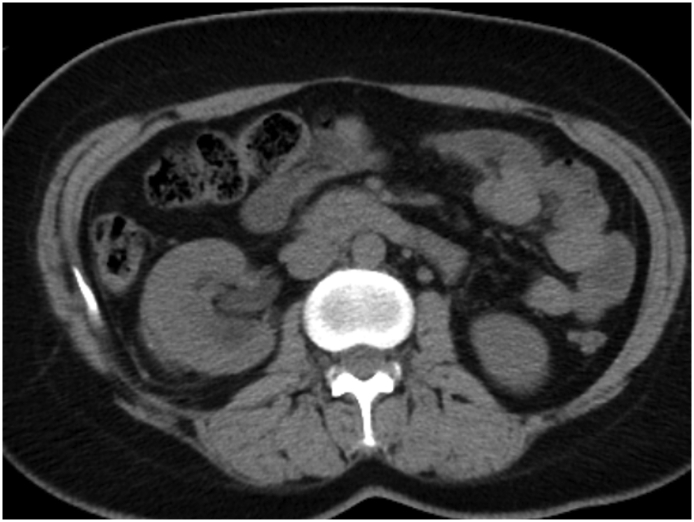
CT showed no recurrence of the renal cyst.
